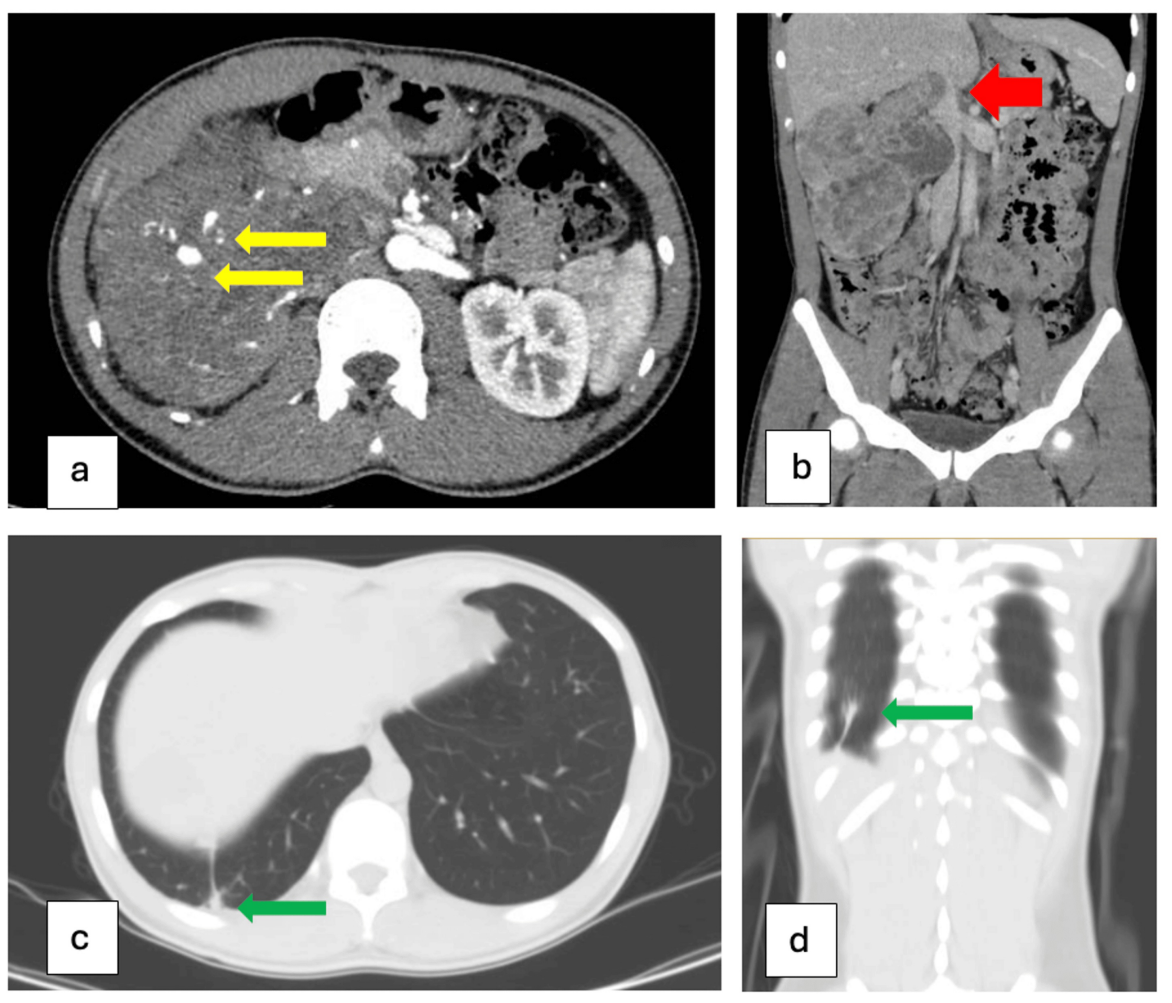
Preoperative MSCT imaging of right renal mass with IVC tumor thrombus and a pulmonary node. (a) Axial arterial phase CT demonstrates a large, heterogeneously enhancing right renal mass measuring 94×126×151 mm, with evidence of perinephric fat invasion. Yellow arrows highlight intratumoral pseudoaneurysms. (b) Coronal portal venous phase CT image demonstrates the tumor exerts a mass effect on the adjacent IVC, resulting in compression and displacement. A contiguous, enhancing soft tissue component within the IVC is visualized, consistent with a tumor thrombus extending from the renal mass (red arrow). (c) Axial chest CT reveals a consolidation lesion in segment 10 of the right lung (green arrow), measuring 25×15×16 mm, suspicious for a benign node. (d) Coronal CT of the chest confirms the location of the pulmonary lesion in the posterior basal segment of the right lower lobe (green arrow). MSCT: multislice computed tomography; IVC: inferior vena cava.
